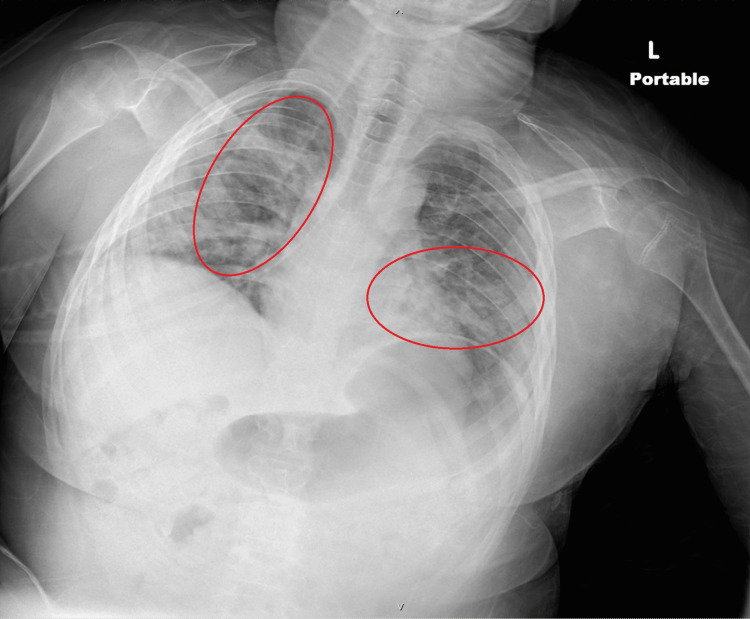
Chest X-ray showing bilateral low lung volumes and patchy pulmonary parenchymal opacities (red circles)
